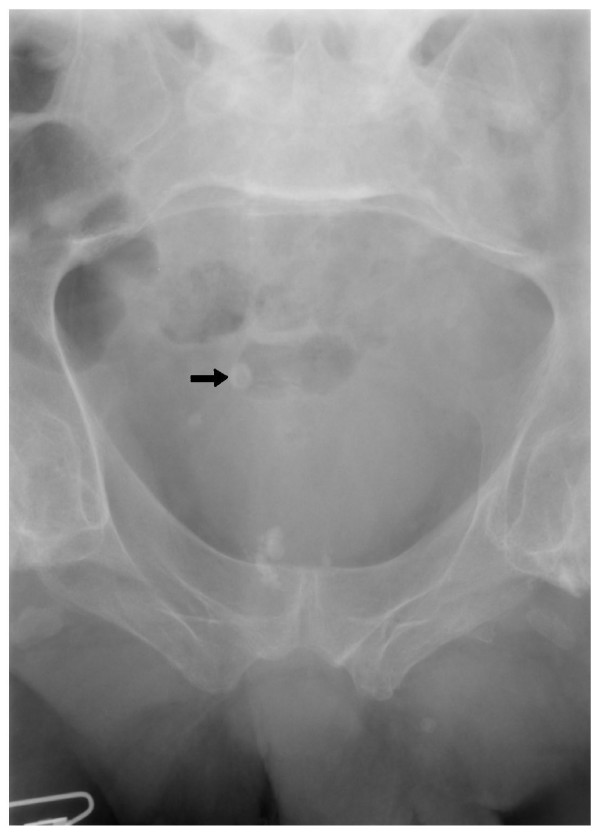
X-ray of urinary bladder taken on 07 March 2005 shows prostatic calcification. There is a radio opaque shadow in the region of urinary bladder, which is probably a vesical calculus (arrow).
